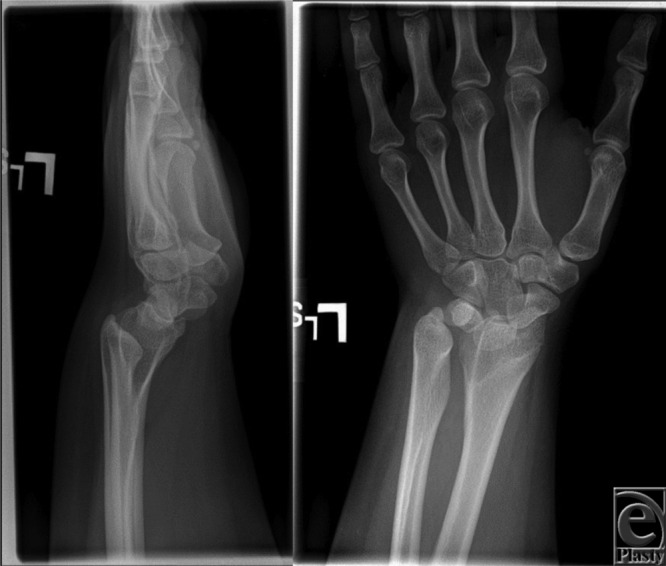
Patient's plain radiographs of the left wrist.
